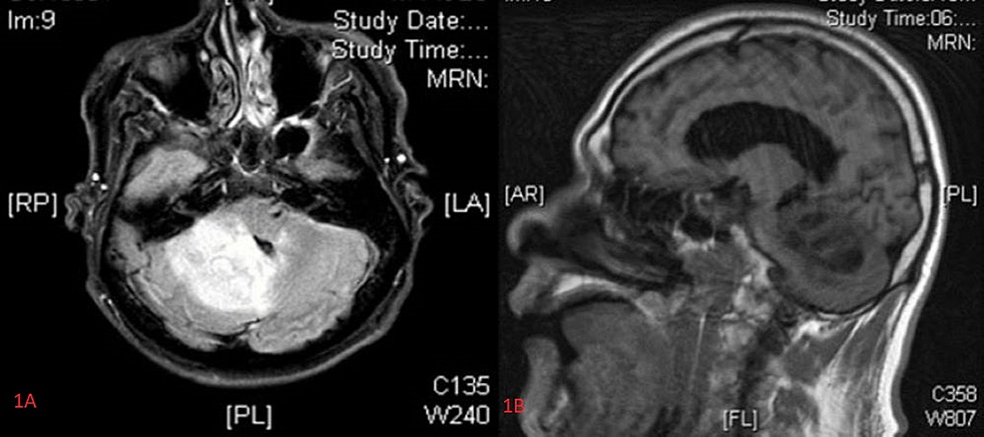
Magnetic resonance images of the cerebellum (T1 mode). Axial and sagittal images showing a cerebellar mass.
